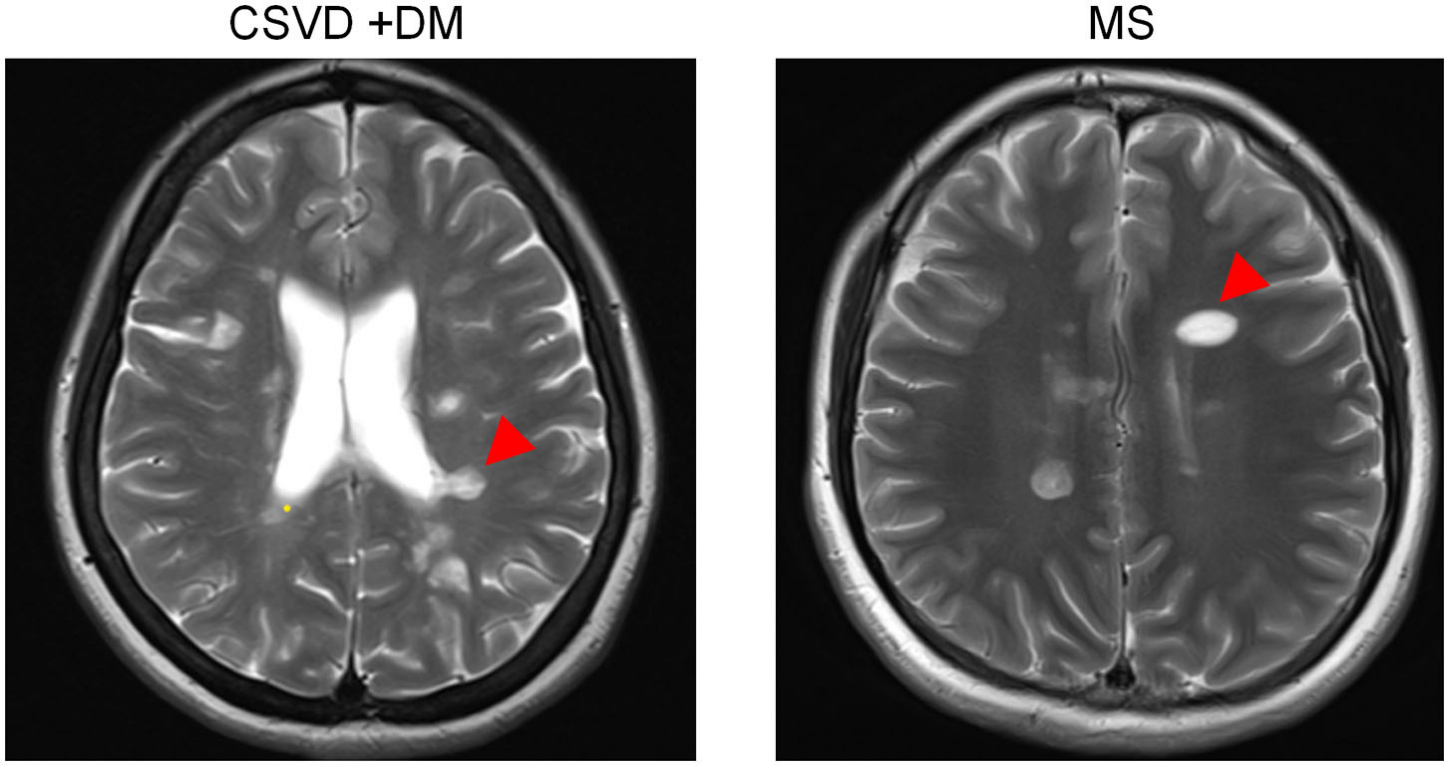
Dawson's fingers of cerebral small vessel disease (CSVD) patients with diabetes mellitus (DM). The venous abnormality feature Dawson's fingers around the ventricles in the fluid attenuated inversion recovery (FLAIR) of both multiple sclerosis (MS) and CSVD patients (arrowheads). Dawson's fingers can be used as a neuroimaging marker of MS and can distinguish between CSVD and MS, but the utility of this marker decreased in patients with DM.
